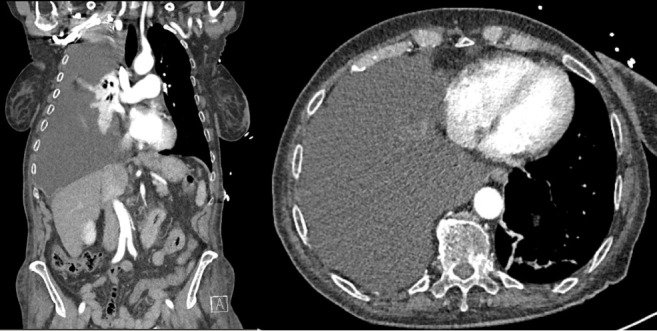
CT chest with unilateral pleural effusion.
